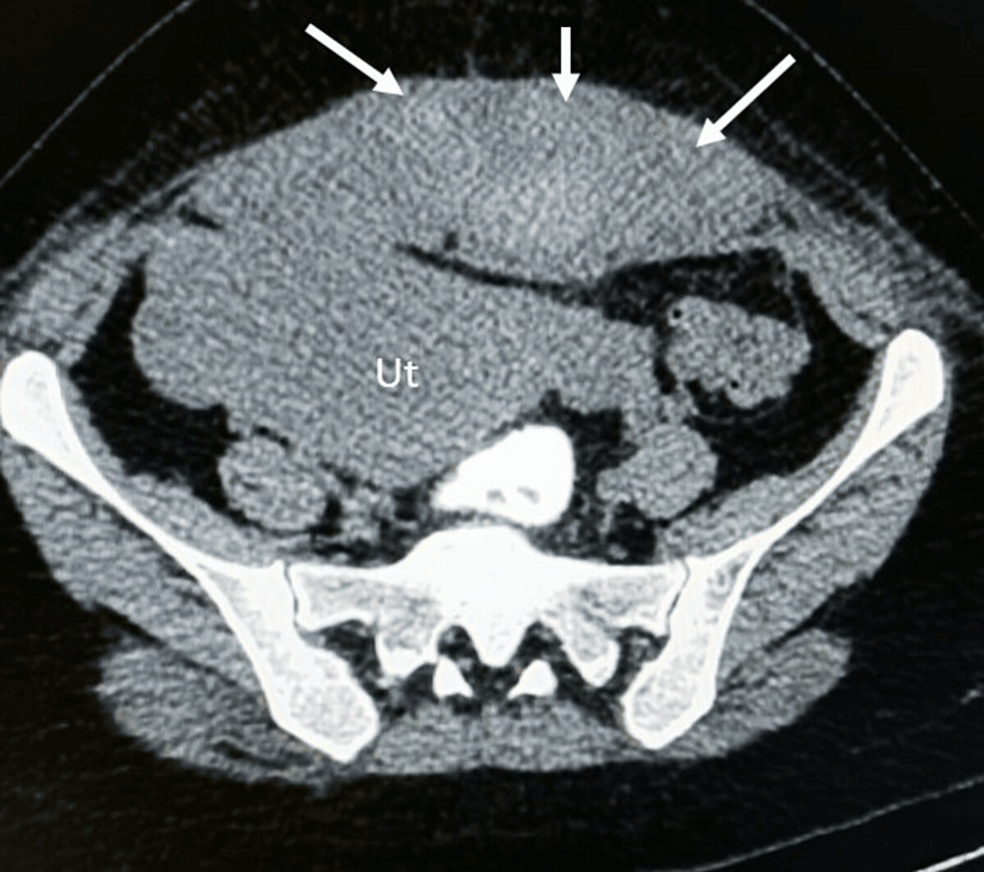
CT scan images of a 30-year-old female showing a large anterior wall hematoma (arrows) measuring 5.2 x 14 x 15.1 cm and extending bilaterally, splaying the abdominal wall muscles. The underlying bulky uterus is denoted by Ut.
